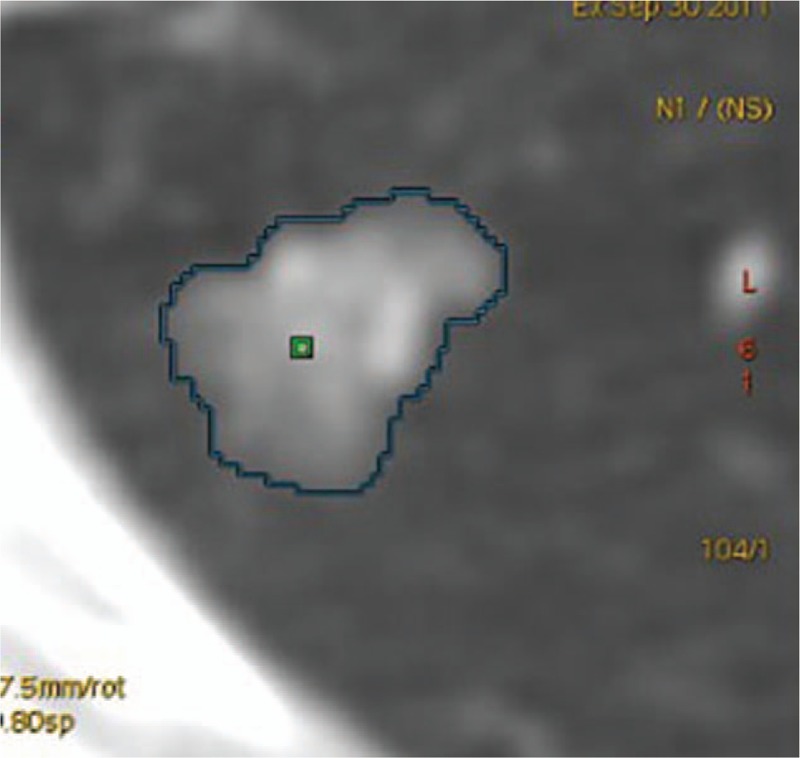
The lesion CTV was measured through Lung VCAR software. CTV = computed tomography volume, Lung VCAR = Lung Volume Computerized Assisted Reporting.
